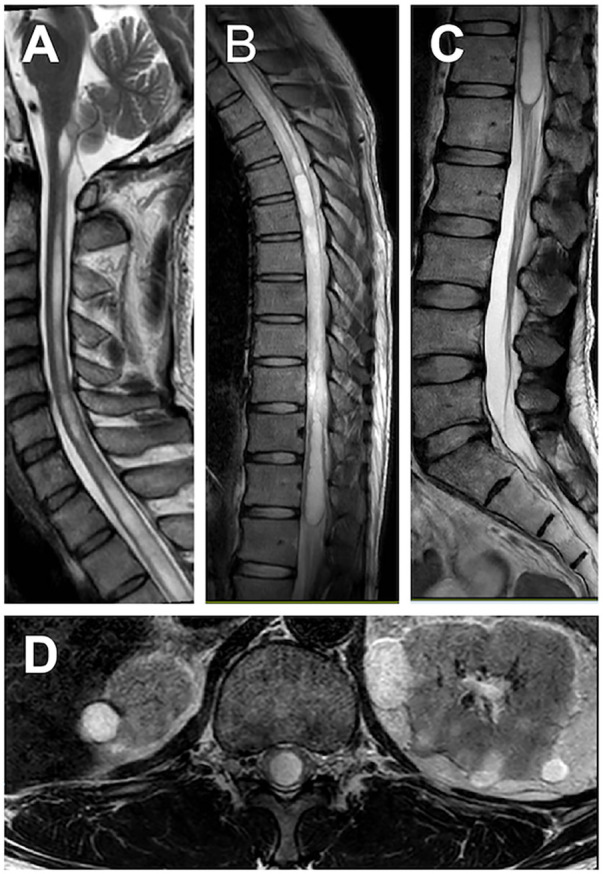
Spinal MRI of Case 4 demonstrating a holocord syrinx involving the entire spinal cord (A–C). Axial MRI at the T12–L1 level showing the maximal diameter of the syrinx (D).
